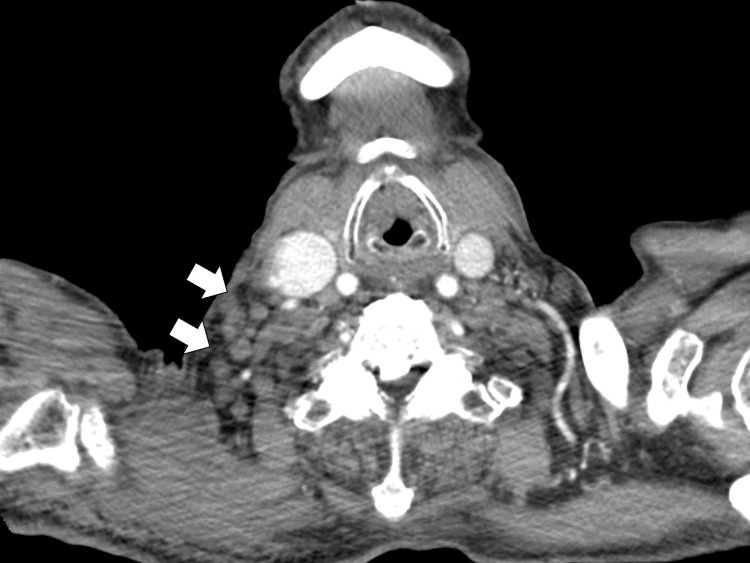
Contrast-enhanced chest computed tomography revealed multiple small lymph nodes in the right supraclavicular fossa (white arrows)
